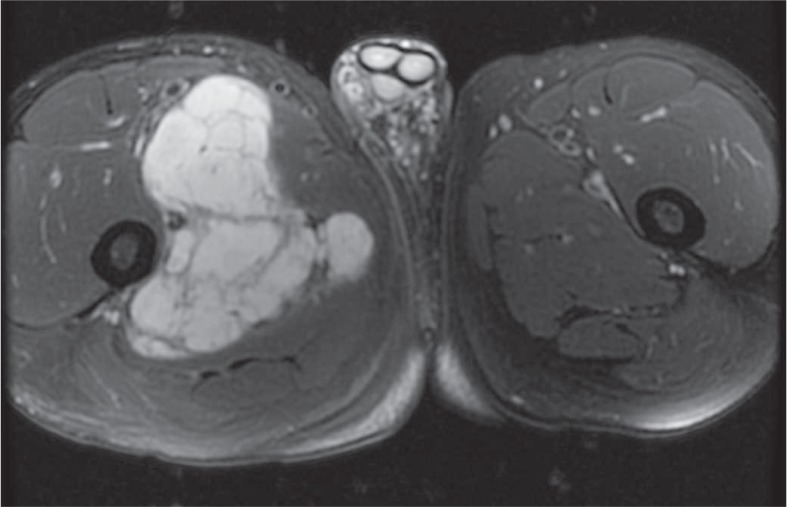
64 year old male with slow growing extraskeletal myxoid chondrosarcoma. Axial T2 fat-saturated (FS) MRI demonstrates a large lobulated T2 hyperintense mass with T2 hypointense internal fibrous septa.
